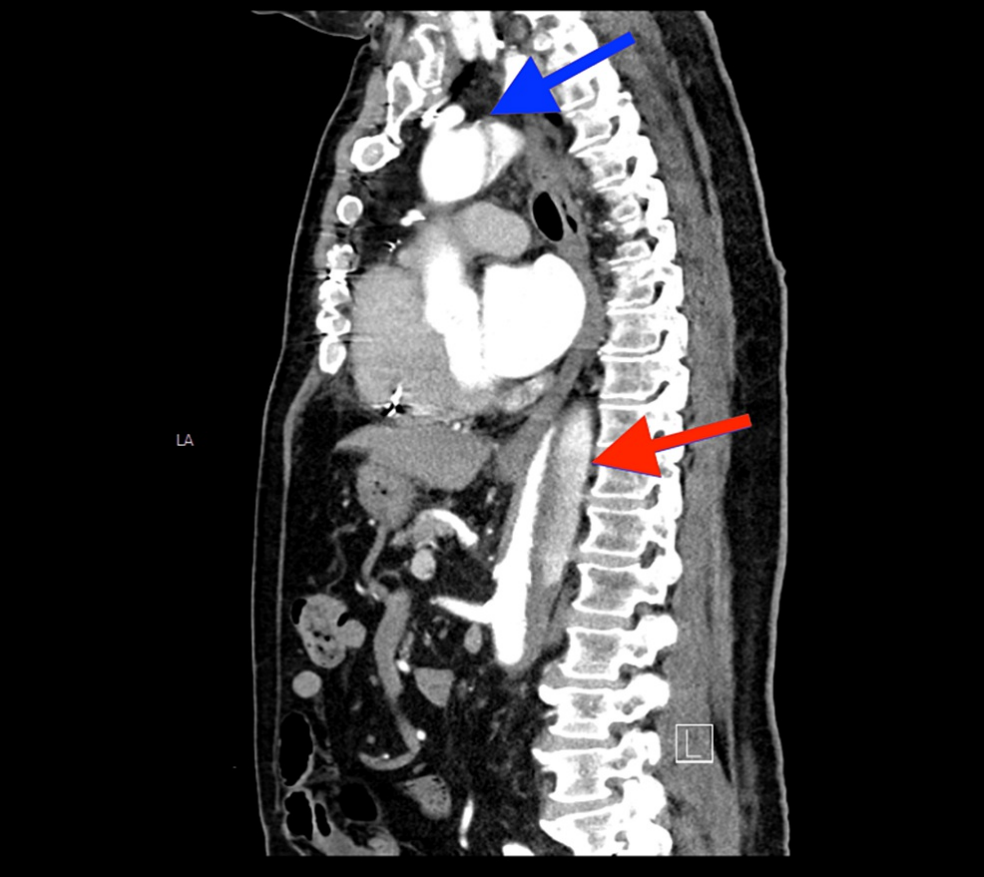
CTA of the chest and abdomen revealing dissection of the ascending (blue) and descending (red) aorta CTA: computed tomography angiography
